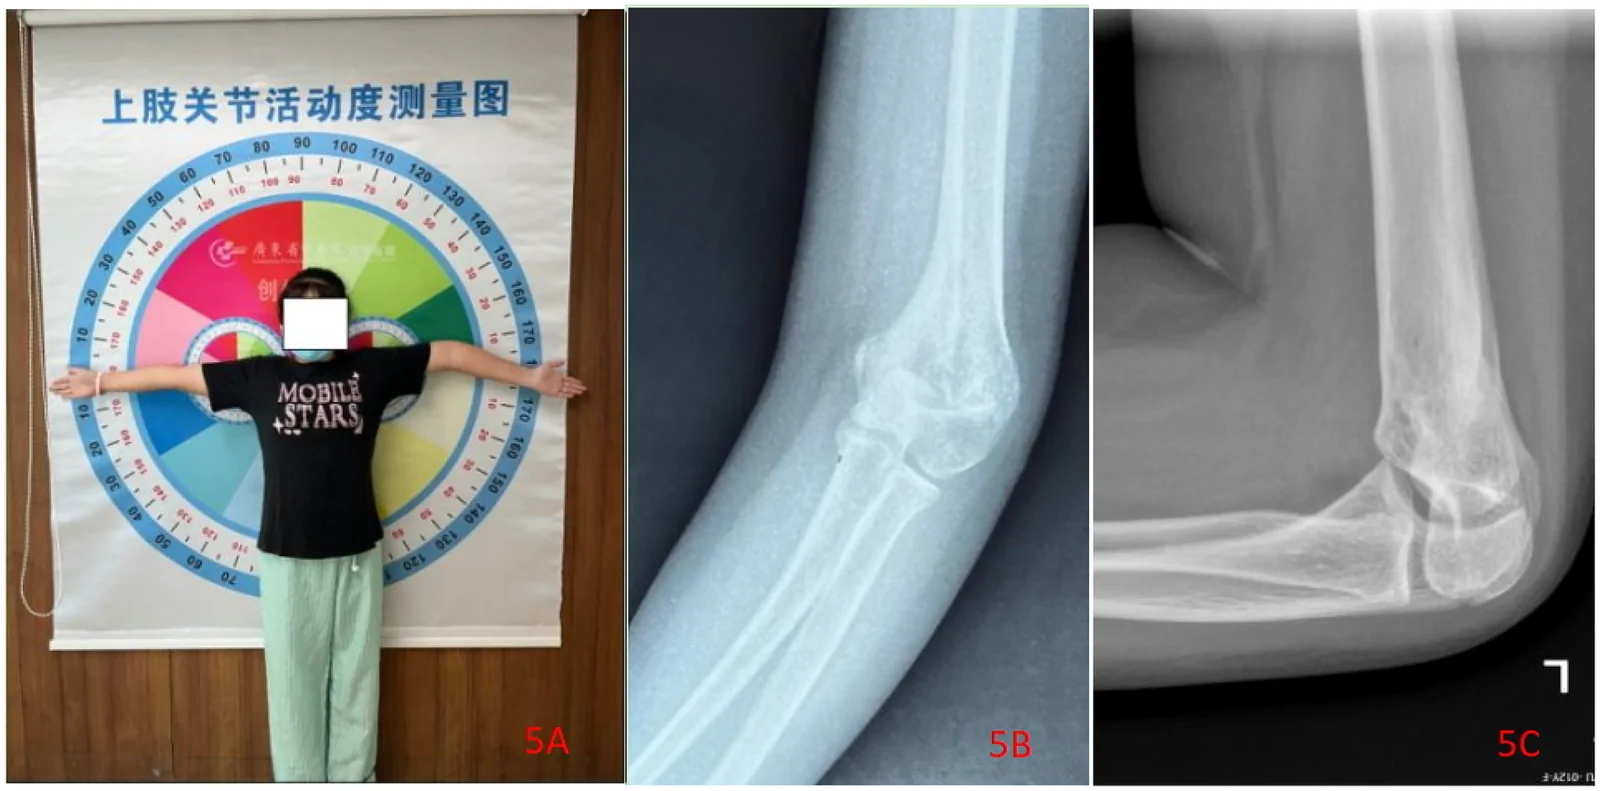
Preoperative presentation of left cubitus varus deformity in a 12-year-old girl. (A–C) Clinical photographs and radiographs showing varus deformity.
